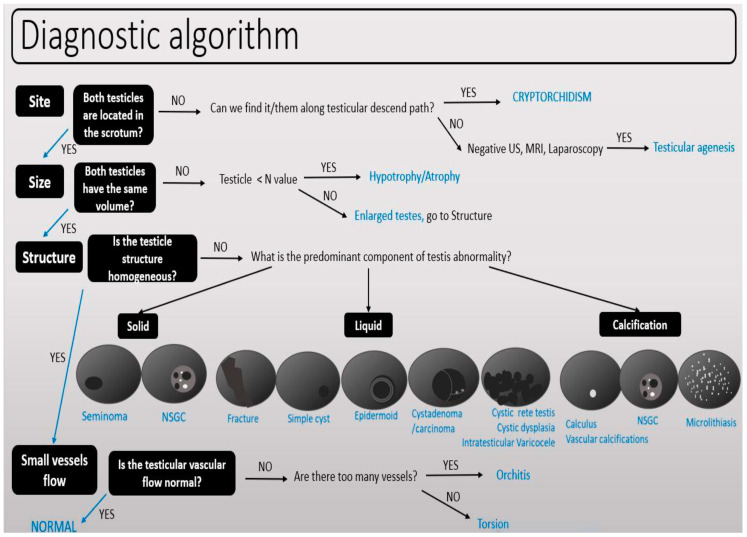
The 4Ss proposed ultrasound imaging algorithm for testicular pathology.
